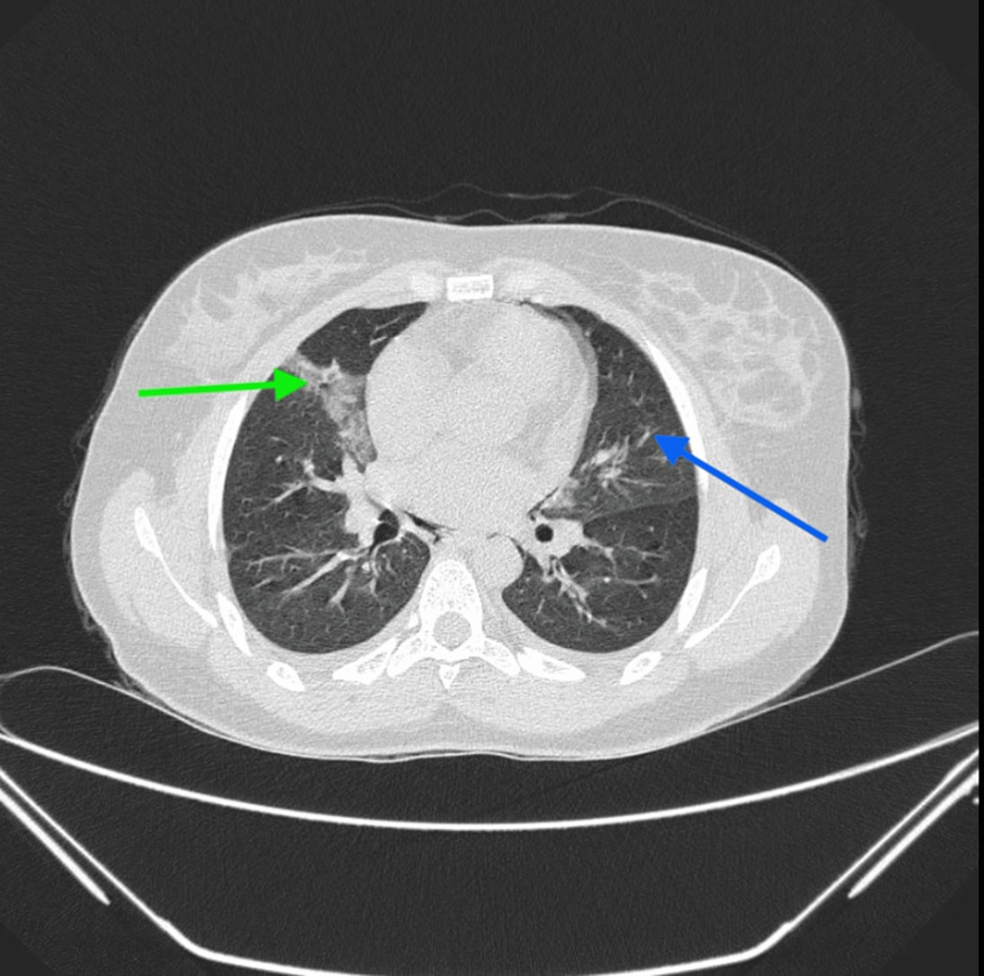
High-resolution computed tomography (HRCT) thorax with ground-glass opacities in the anterior and medial basal segments of the right lower lobe (green arrow) and the anteromedial segment of the left lower lobe (blue arrow)
